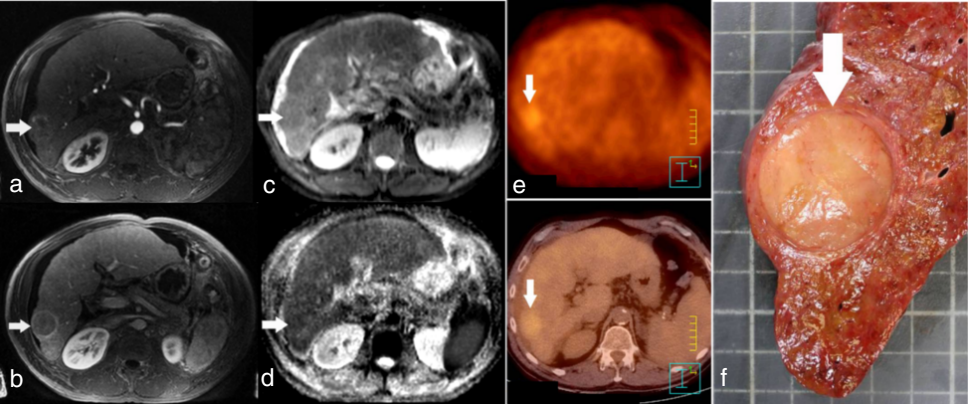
Poorly differentiated HCC in a patient with alcoholic cirrhosis, showing restricted ADC on DWI-MRI and increased uptake on the FDG-PET/CT. Transverse dynamic contrast-enhanced initial post contrast MR image shows a 30 mm large nodular enhancing lesion in the right liver on 3D GE arterial phase imaging (a, *arrow*) with wash-out on portal-venous phase (b, *arrow*). The lesion is mildly hyper intense on transverse DW image (b = 800 s/mm^2^; c, *arrow*) and has a low ADC (1.06 × 10^−3^ mm^2^/s) on the ADC map (d, *arrow*). FDG-PET/CT shows an increased tumour uptake with a SUV_T_ = 4.1 and SUV_T/L_ = 1.24 (e, *arrow*). The explanted liver showed an Edmondson-Steiner grade 4 HCC (f, *arrow*)
